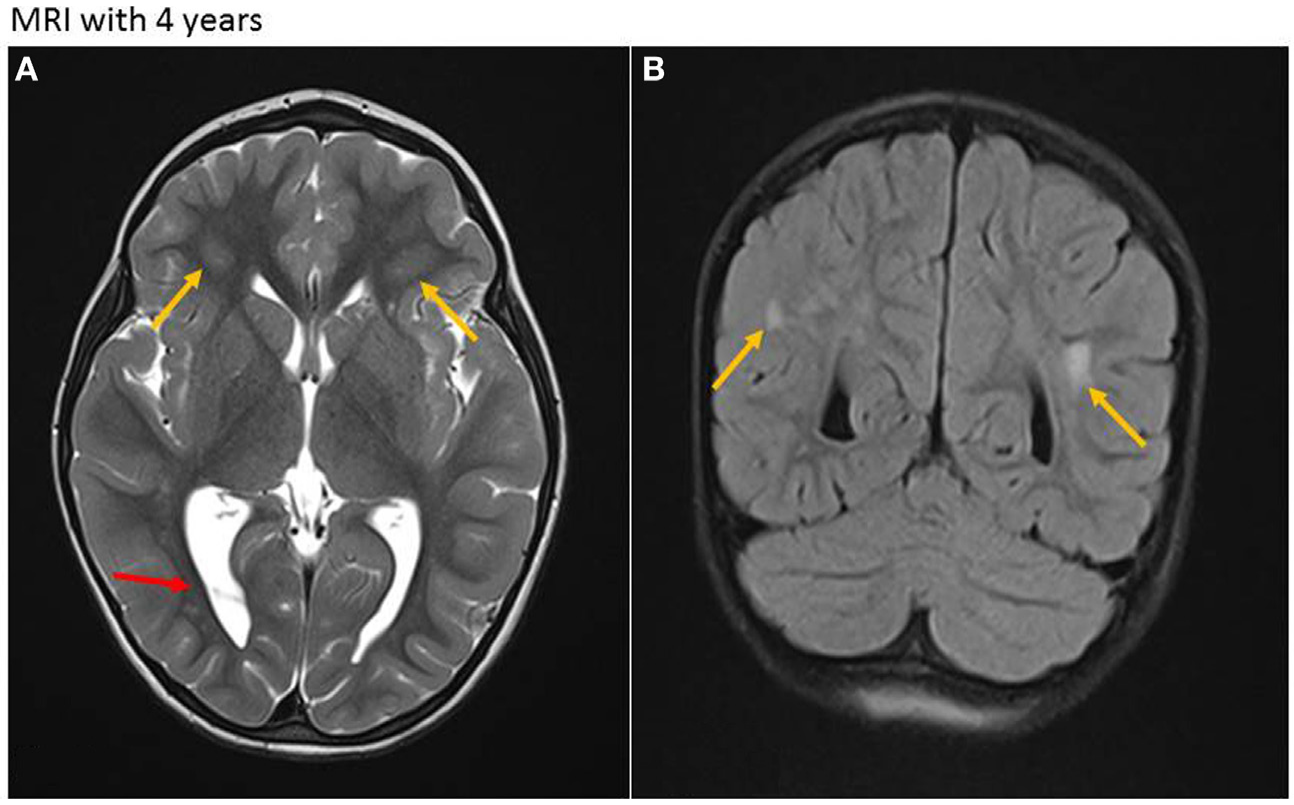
Magnetic resonance imaging (MRI) at age of 4 years. (A) Axial T2-weighted sequence. Residual white matter hyperintensities (due to demyelination and gliosis) with periventricular location on both sides (yellow arrows) and persistent intraventricular septa, mainly in the occipital region (red arrow). (B) Coronar T2-weighted sequence. Residual periventricular hyperintense white matter lesions (yellow arrows).
